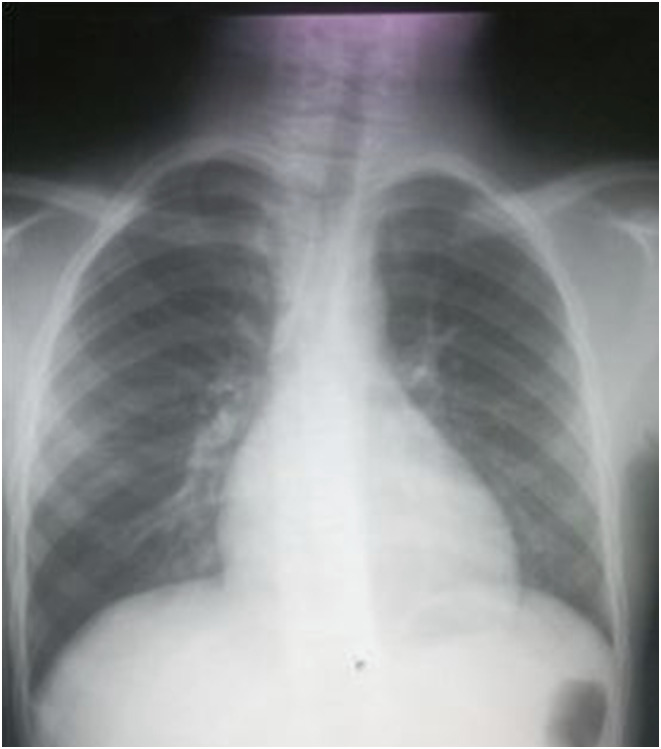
Chest X-ray of patient 1, which had right radio sternal lymph node enlargement.
